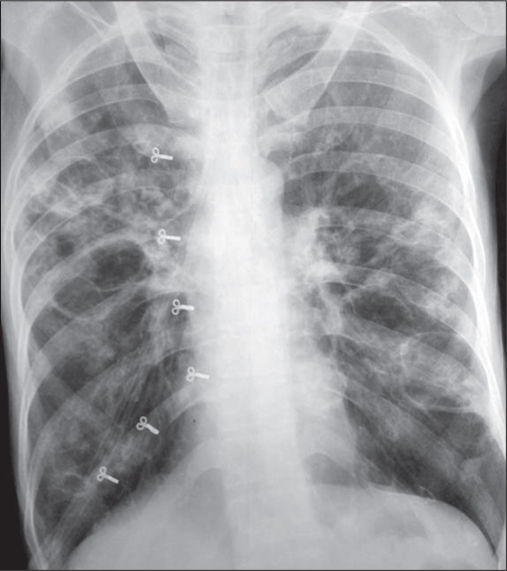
Chest X-ray PA view at 4 weeks showed clearing of the opacities with thin-walled cavities.
